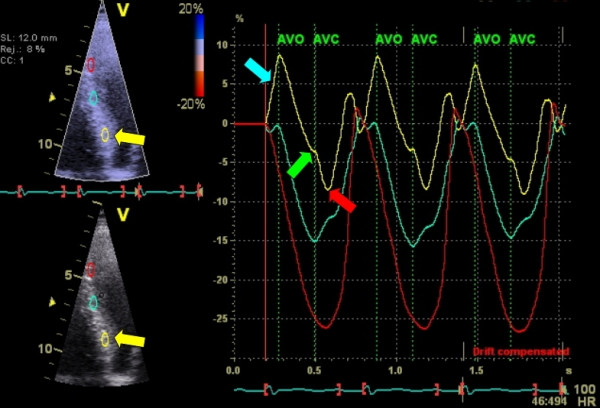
Tissue Doppler derived strain curve of three cardiac cycles of a patient suspected for HCM. Yellow-curve/arrow = basal segment, cyan = mid, red = apex. Note the abnormal deformation pattern in the yellow curve. There is prestrech (blue arrow), a reduced peak systolic value (green arrow) and post-systolic shortening (red arrow).
